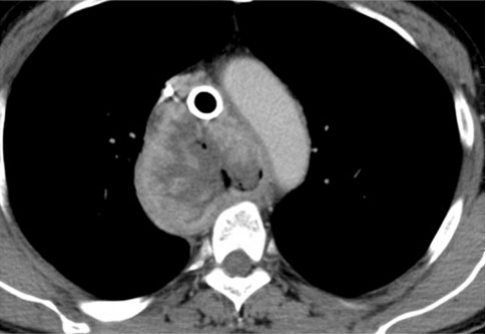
Chest computed tomography (CT) shows a huge esophageal tumor significantly compressing the trachea with the stent
